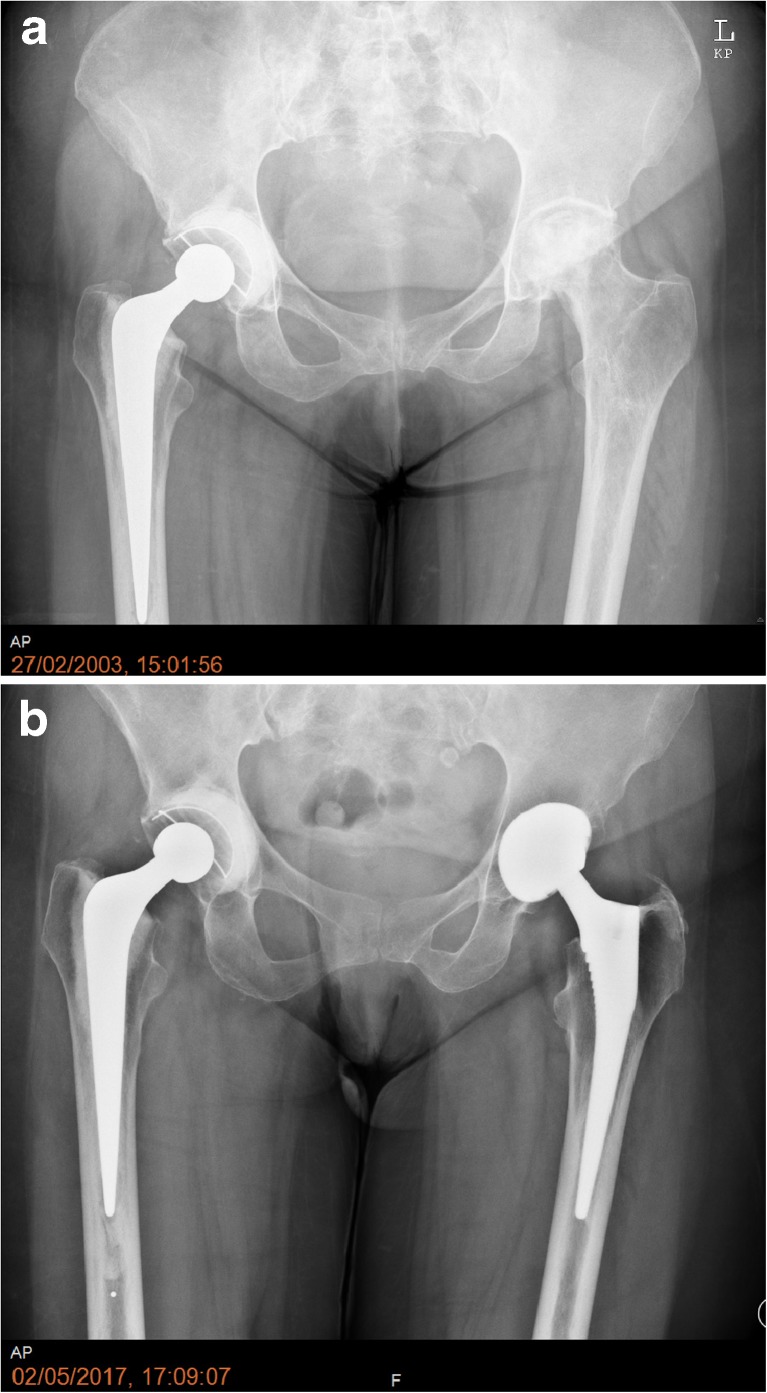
a One-year post-operative radiographs of a 45-year-old female with a cemented Exeter THA and pre-operative left side with Steinberg stage 5 ON. b Fifteen-year post-operative radiographs right side with minimal eccentric polyethylene wear and 14-year post-operative left uncemented THA
